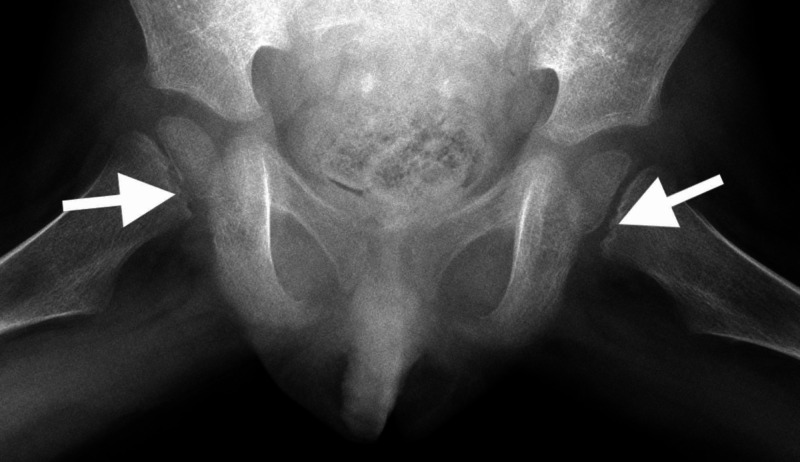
X-ray of the pelvis (frog leg lateral) from third presentation to hospital (arrows pointing to subcortical osteoporotic rings within both femoral capital epiphyses)
